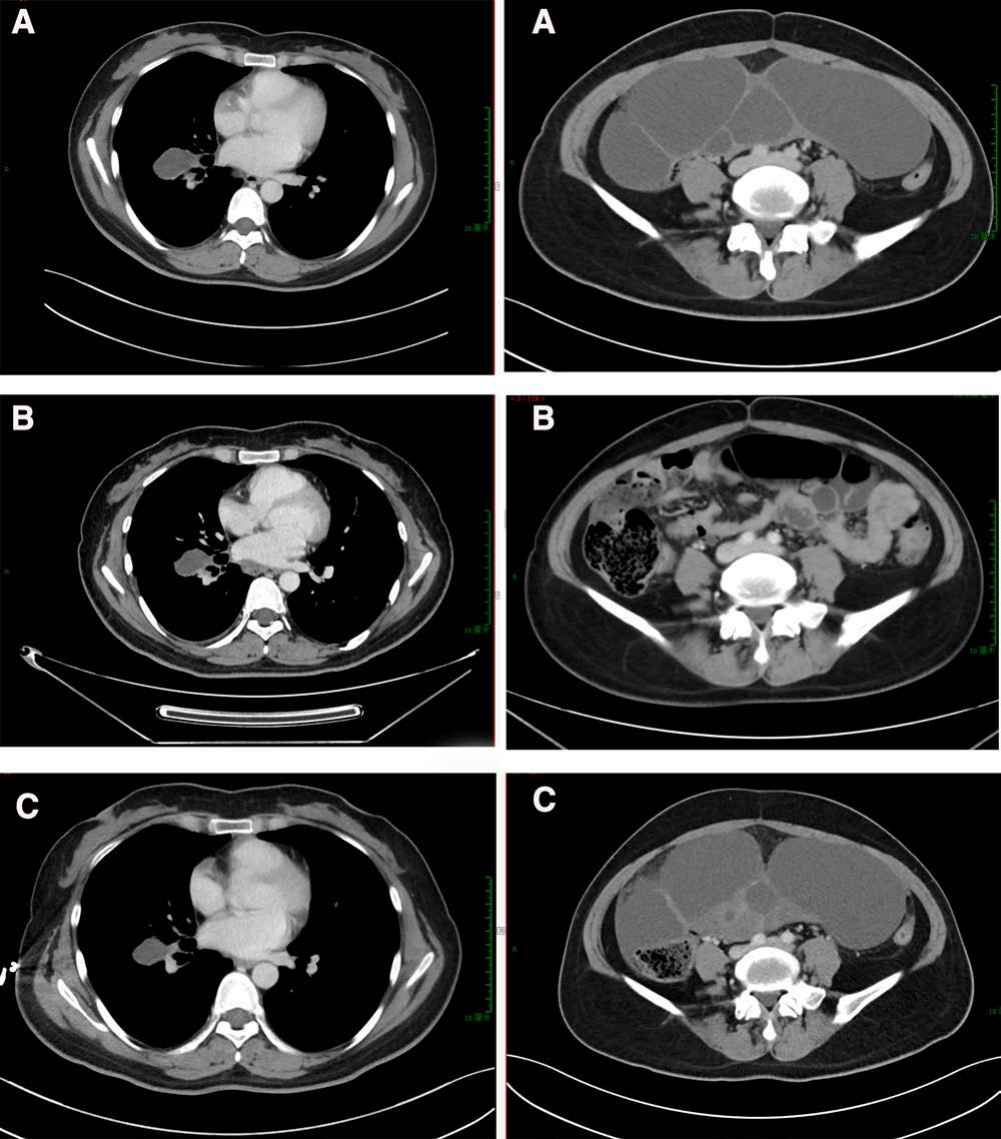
Computed tomography: February 25, 2022; May 23, 2022; and July 4, 2022. (A) May 25, 2022, cross-section of lung and pelvic tumors. (B) May 23, 2022, cross-section of lung and pelvic tumors. (C) July 6, 2022, cross-section of lung and pelvic tumors.
